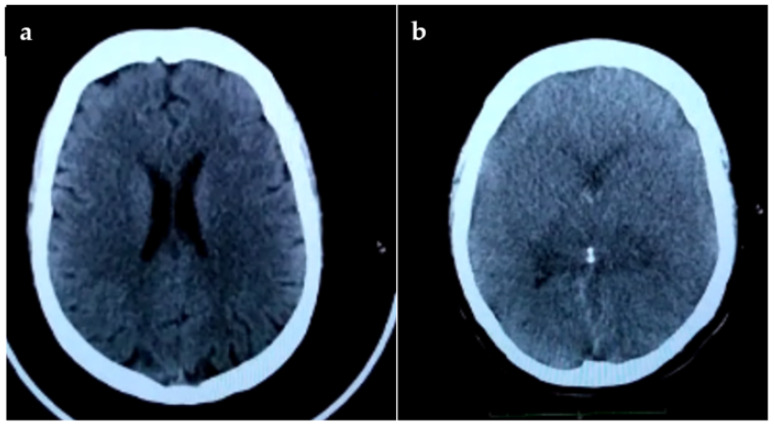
(a) Computed tomography does not show significant changes in the cerebral ventricles; (b) This image demonstrates frank cerebral edema with collapse of the ventricles and effacement of the fissures.
